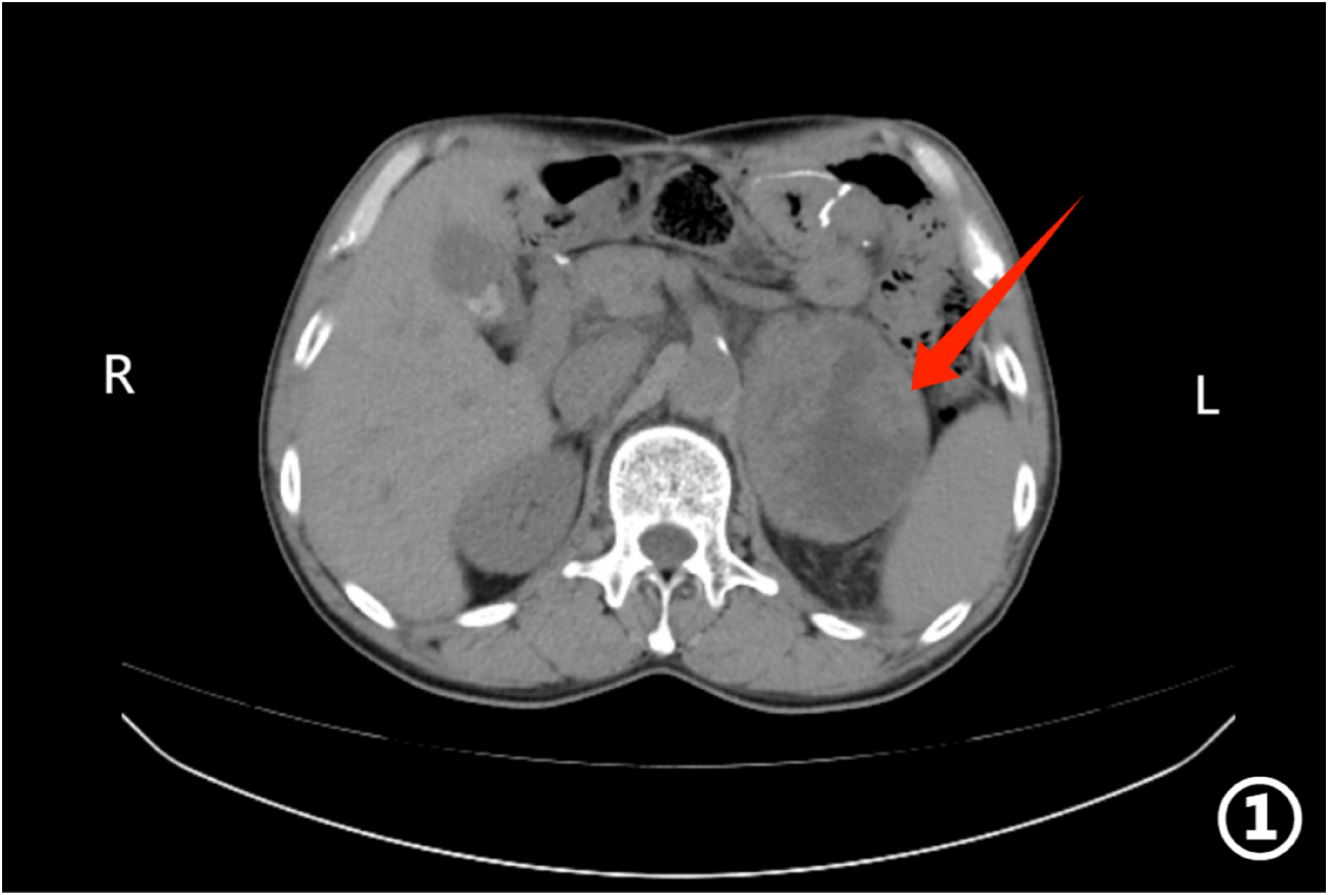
Axial abdominal CT image showing a large area of low-density shadow in the left upper abdomen within the splenic region (red arrow).
